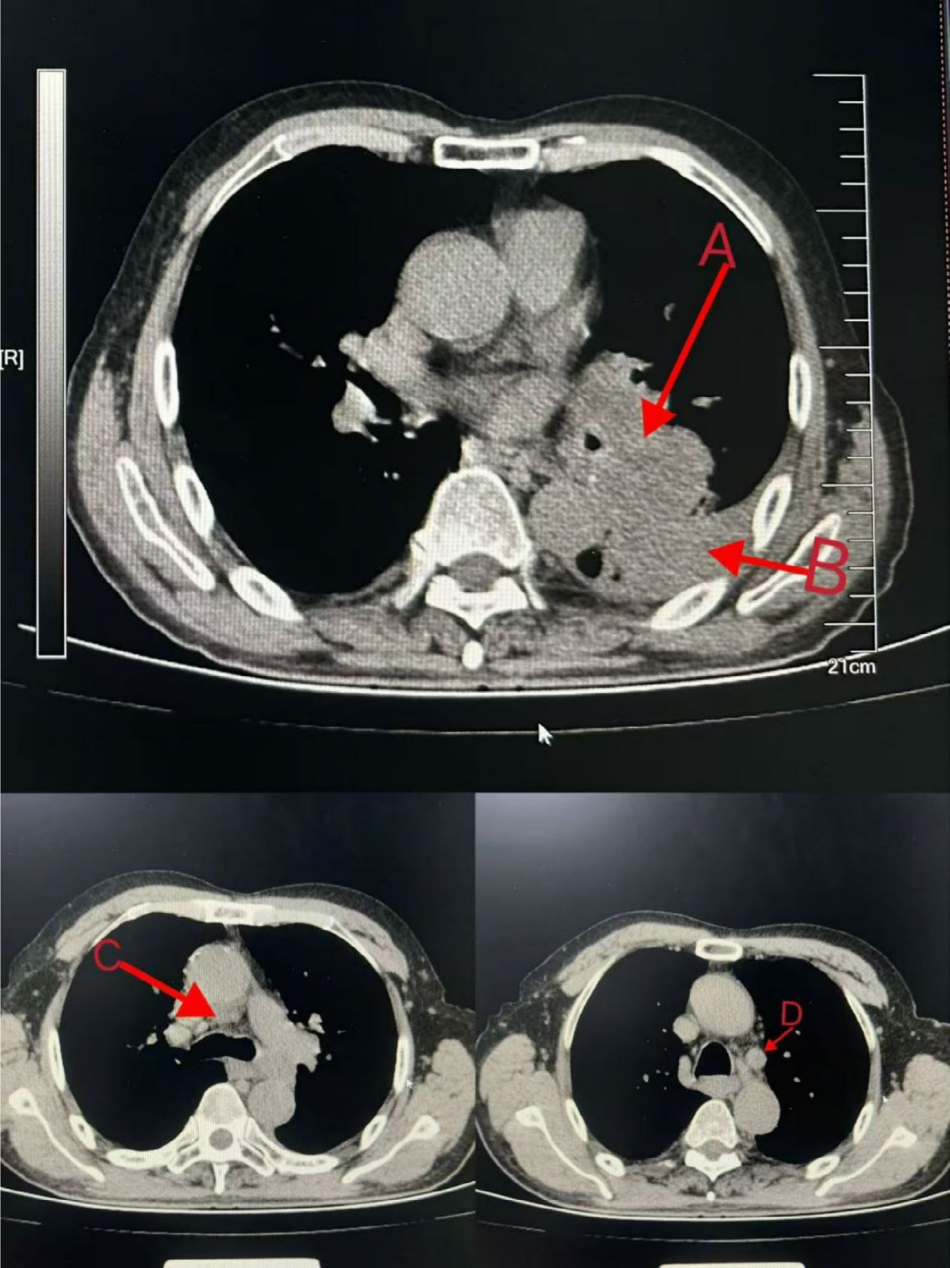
Chest CT identified a left lung mass with pleural (A) and rib invasion, multiple mediastinal (C) and hilar lymph node metastases (D), and pleural effusion (B). (2023/6/22 Chest CT).
